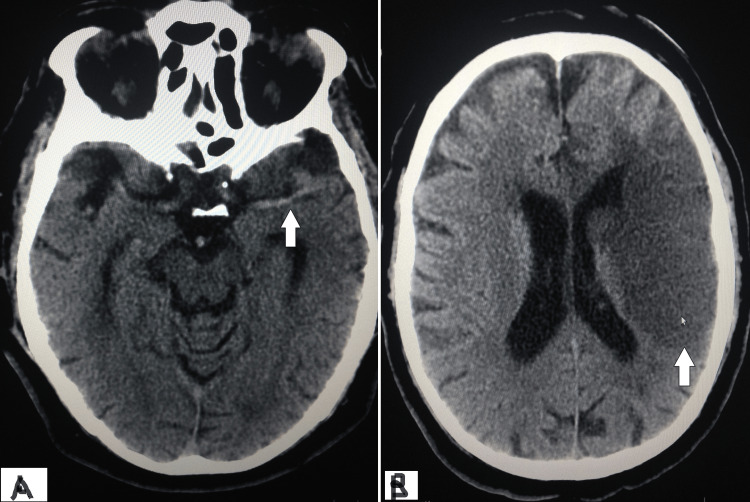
(A) Initial computed tomography (CT) of head showing left side middle cerebral artery as shown with white arrow, dense MCA sign (B) CT head, after 12 hours, shows fully evolved left side MCA territory infarct as depicted by white solid arrow.
